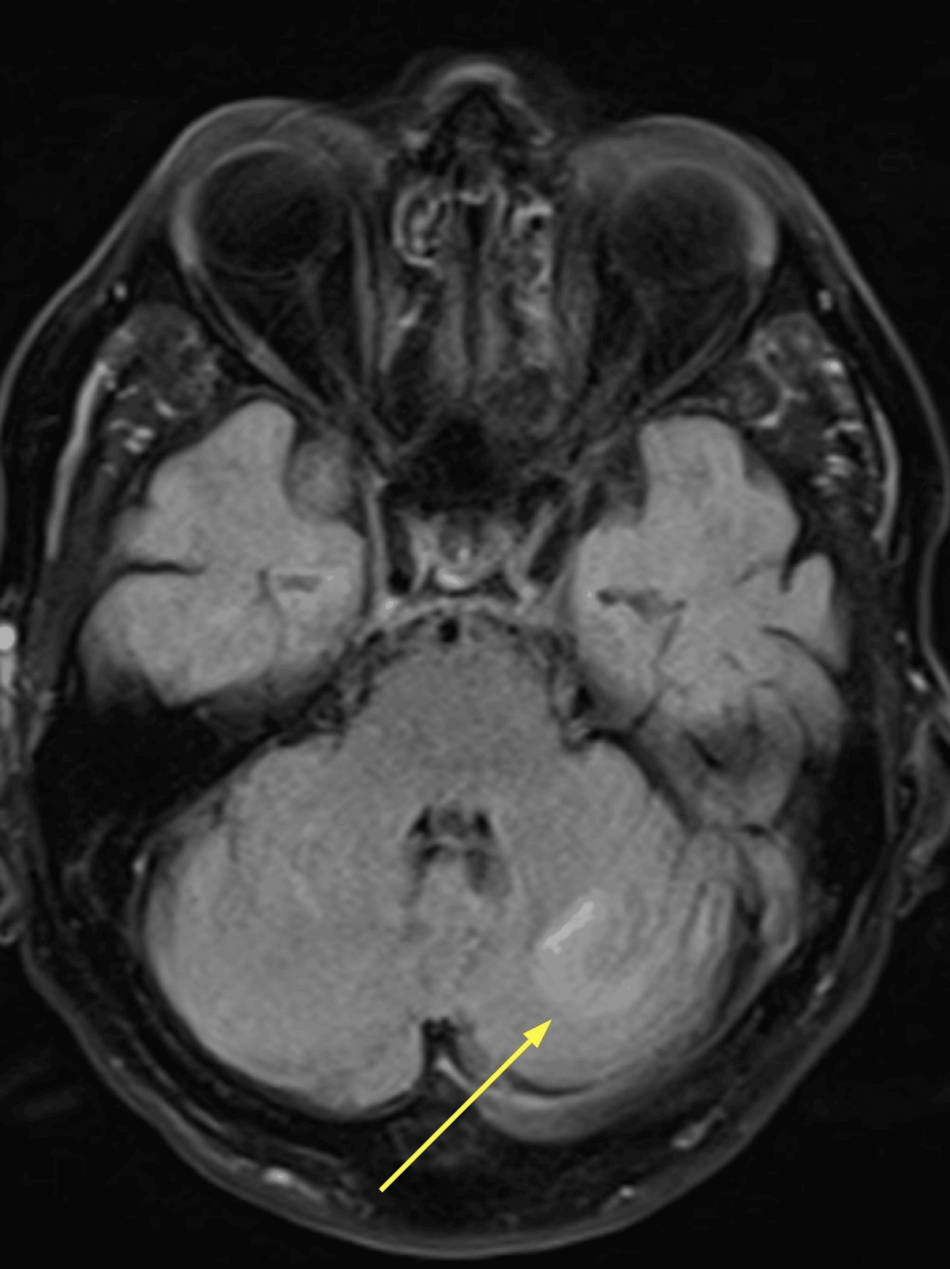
MRI brain: cerebellar section Within the superior right cerebellar hemisphere, a high signal appears to lie predominantly within the CSF spaces between the folia. Within the superior left cerebellar hemisphere is a 2 cm region of parenchymal FLAIR hyperintensity. The yellow arrow is pointing to the lesion in the left cerebellar hemisphere. CSF, cerebrospinal fluid; FLAIR, fluid-attenuated inversion recovery
